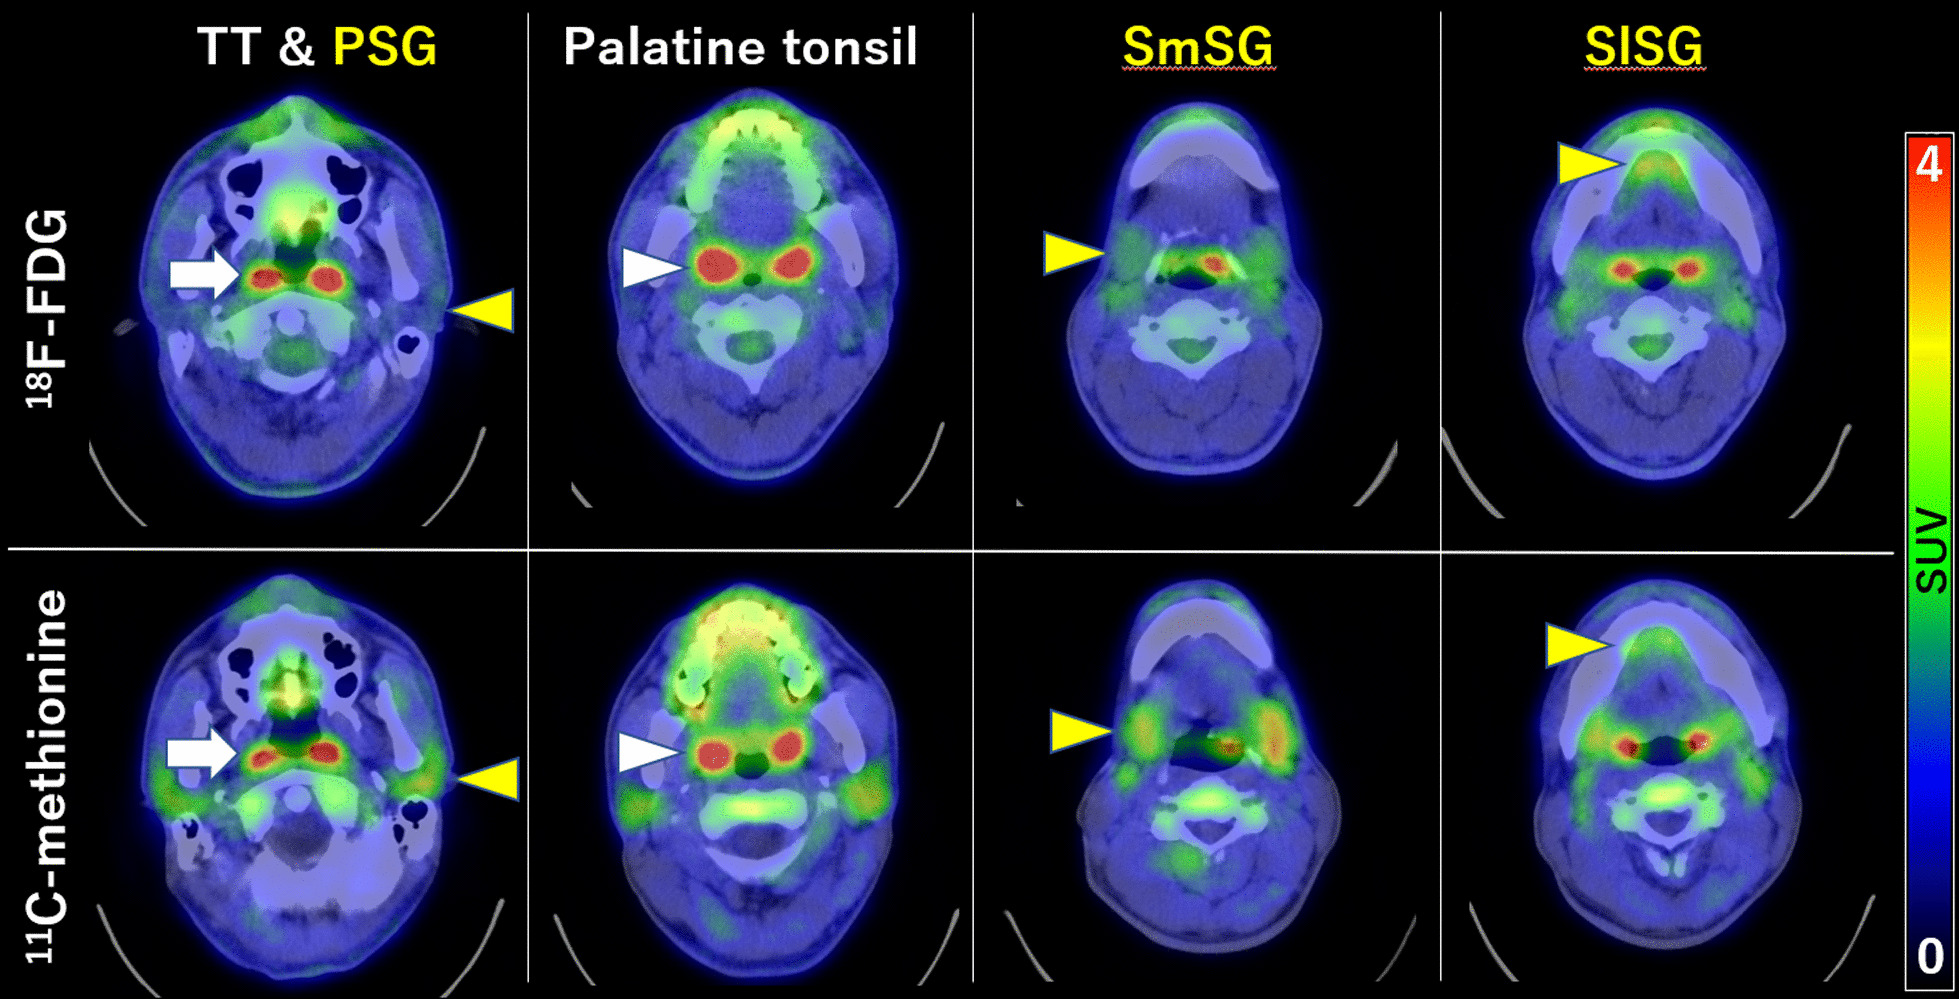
Representative PET/CT images of a 37-year-old male patient. Upper row, [^18^F]FDG PET/CT images; lower row, [^11^C]methionine PET/CT images. TT (tori tubarius, white arrows), Palatine tonsil (white arrowhead), PSG (parotid salivary gland, yellow arrowheads), SmSG, (submandibular salivary gland, yellow arrowheads), and SlSG (sublingual salivary gland, yellow arrowheads)
